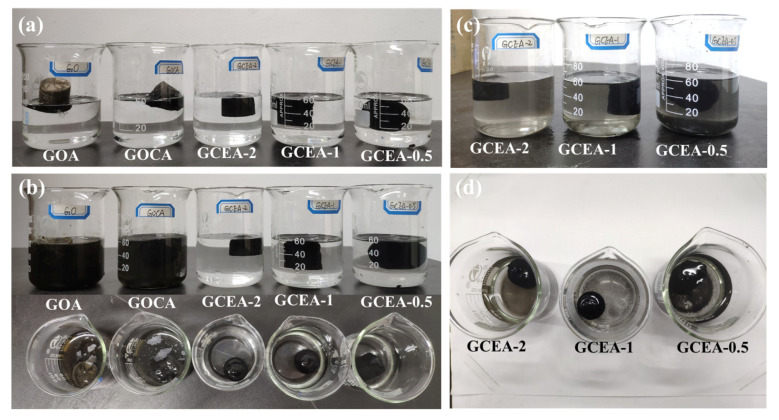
The digital images of GOA, GOCA, and GCEA immersed in water, (a) 0 min, (b) ultrasonic treatment for 1 min (side and top view), (c,d) the GCEA in ultrasonic treatment for 30 min (Color figure can be viewed online).
